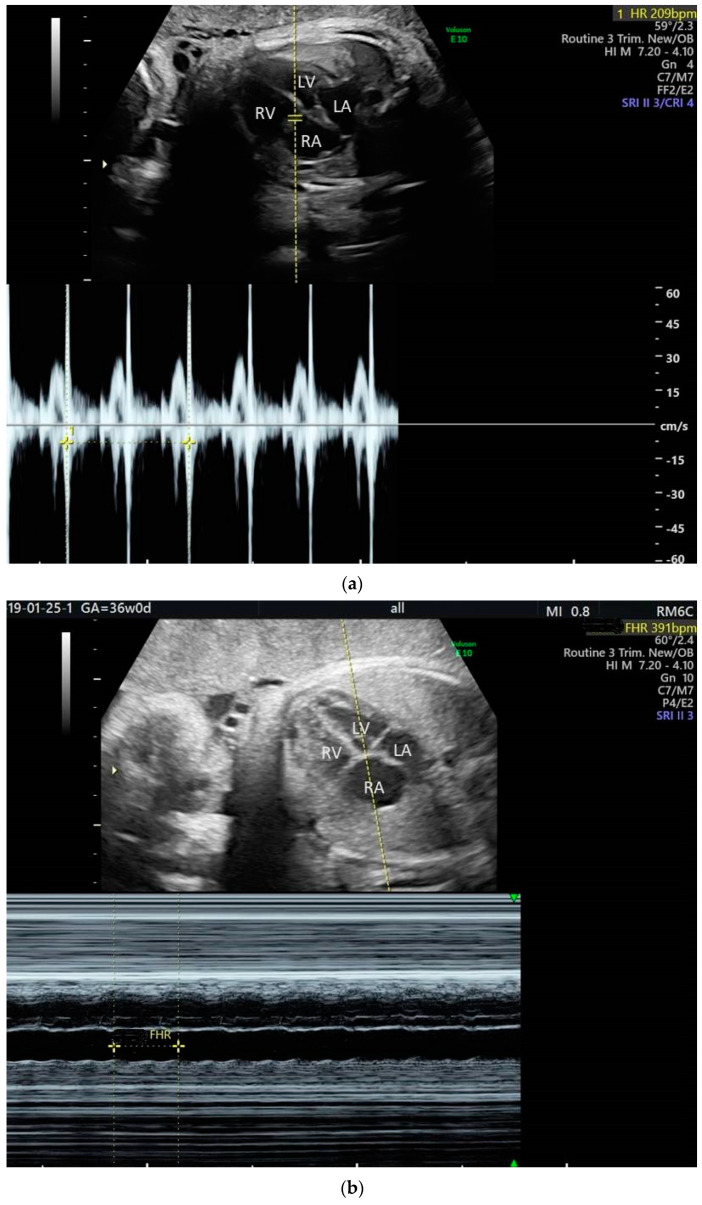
Fetal atrial flutter: (a) Pulsed Doppler analysis—ventricular rate; (b) M-mode analysis—atrial rate.
